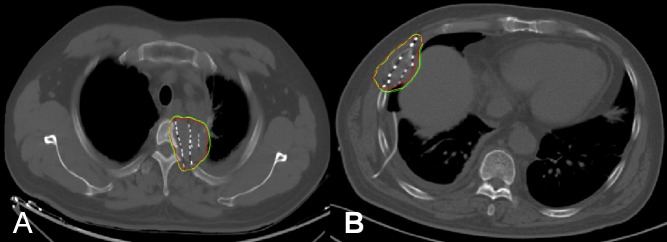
Administration of ^125^I-seed brachytherapy The isodose curve after seed implantation on CT scan. Red lines represent the contour of the tumour. The yellow and green curves are isodose lines of D100 (120 Gy) and D90 (108 Gy), respectively. A. Image of a 61-year-old man with metastatic esophageal cancer involving the thoracic vertebra. B. Image of a 48-year-old man with metastatic non-small-cell lung carcinoma involving the rib.
